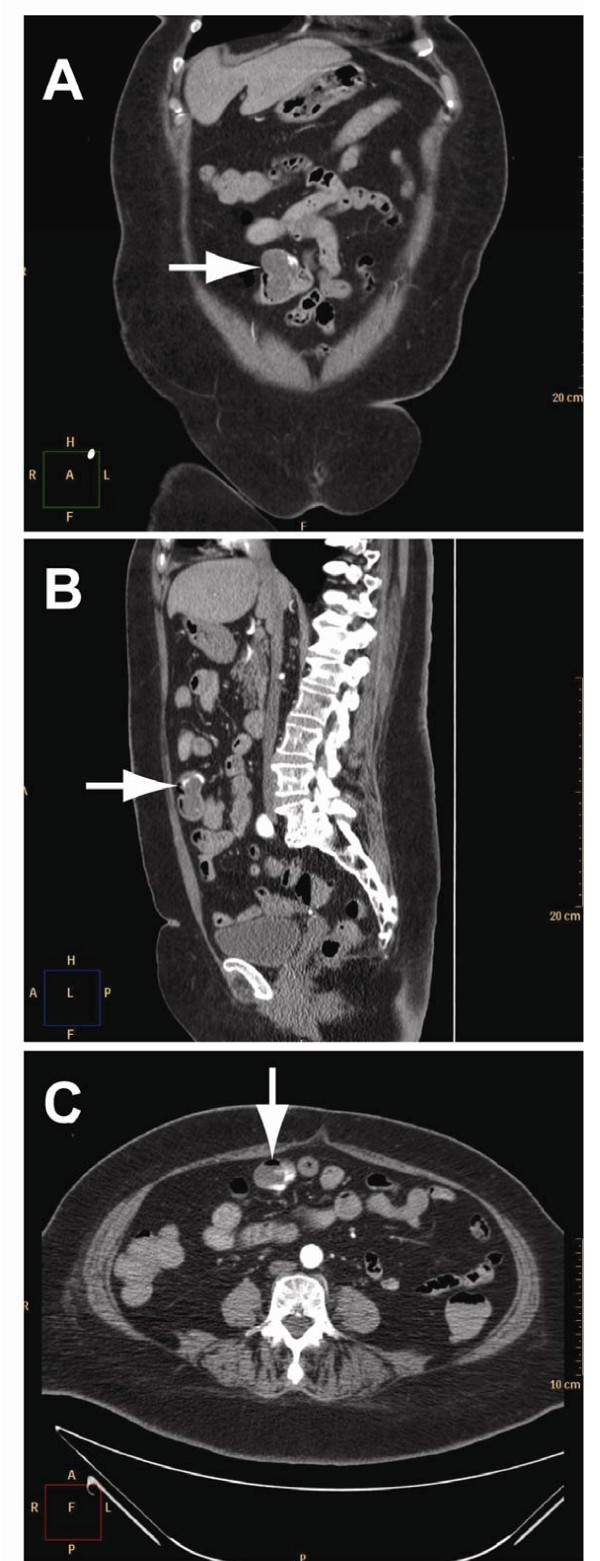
Abdominal computed tomography (CT) angiography in arterial phase. A, Coronal abdominal CT demonstrating contrast extravasation in small intestine diverticulum, diagnostic for bleeding (white arrow). B, Jejunal diverticulum with bleeding seen on sagittal abdominal CT (white arrow). C, The bleeding in jejunal diverticulum demonstrated on axial abdominal CT (white arrow).
